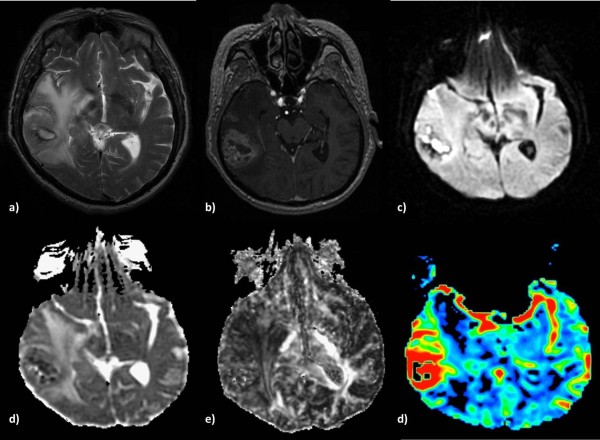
Anaplastic Astrocytoma in a 71-year-old man. a) T2-weighted image shows increased signal intensity with peritumoral edema, b) heterogeneous contrast enhancement on a post-contrast 3D-SPGR image and c) restricted diffusion in the solid portion of the tumor. The lesion is hypointense on the ADC map (d), presents low FA (e) and increased perfusion on the rCBV map (f).
